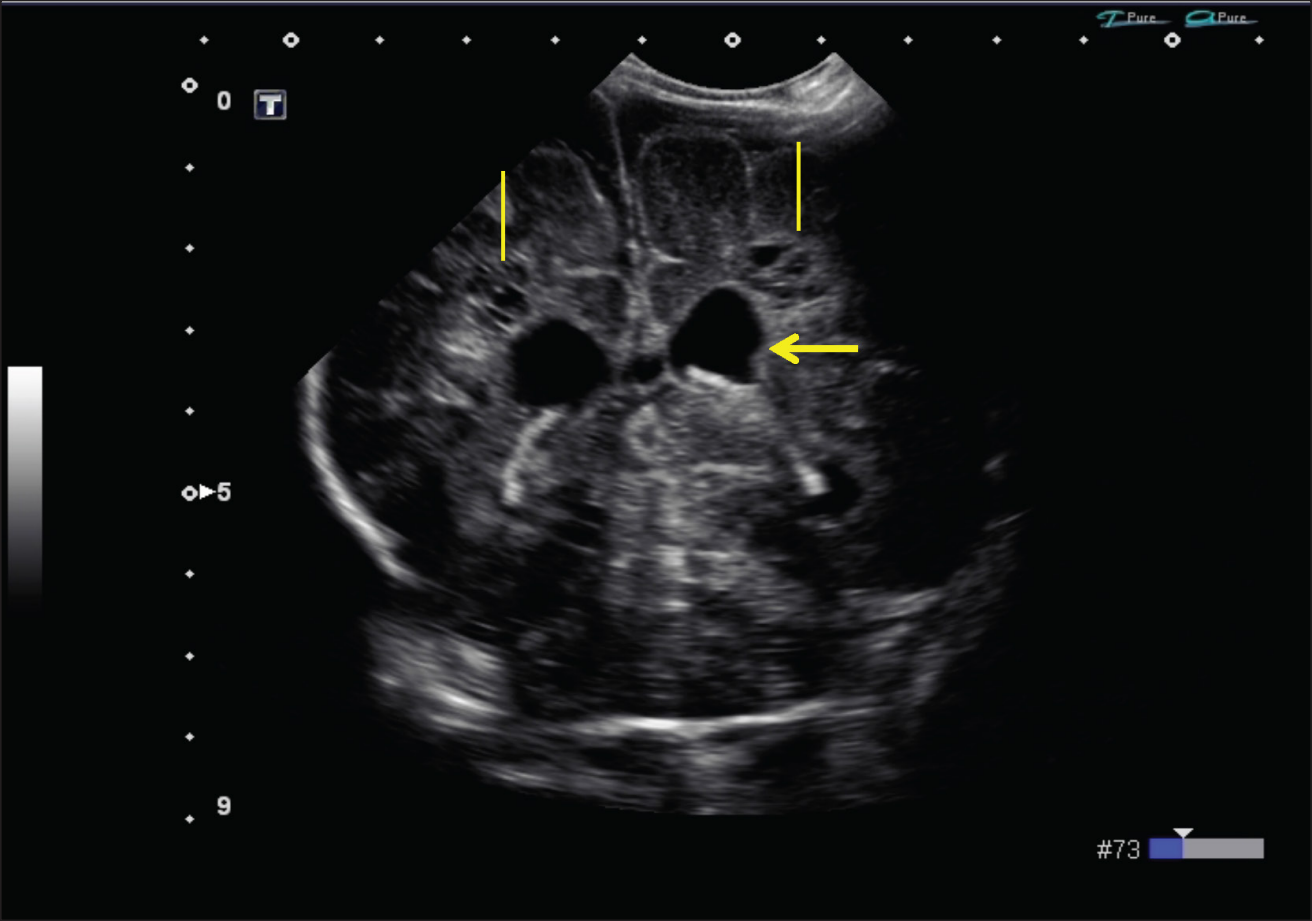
Transcranial ultrasound, 8 weeks post-partum, coronal plane. Progressive enlargement of lateral ventricles (arrow), and formation of periventricular cysts (straight lines).
